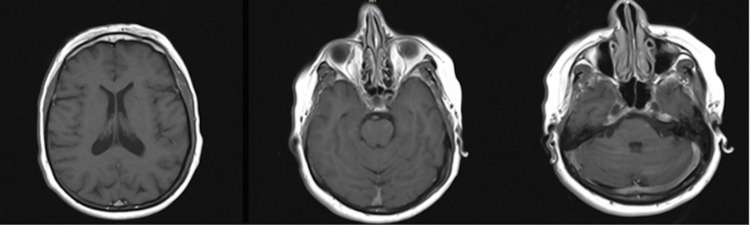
MRI head with contrast. Axial MR images of the brain, with contrast sequences. No evidence of intracranial metastasis, demyelination, or encephalitis was identified. No facial cranial nerve or other cranial nerve pathology was suspected.
